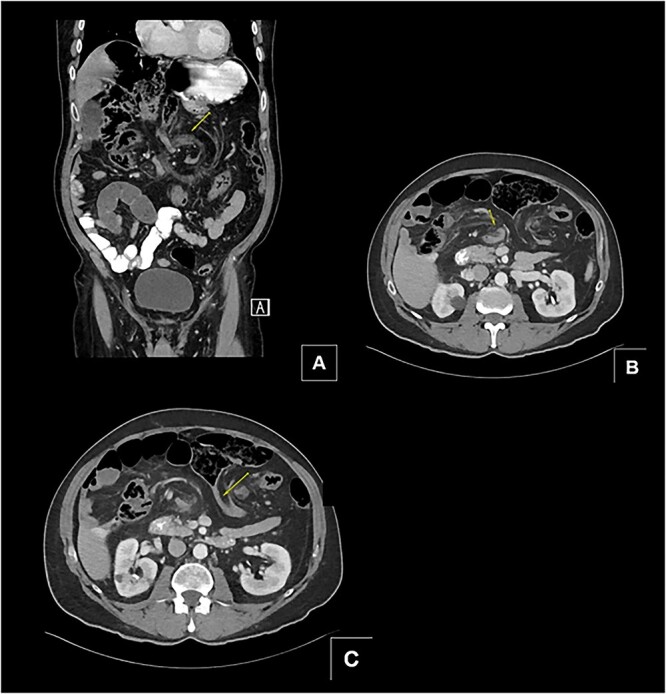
(A and B) Two separate CT images showing mesenteric swirling in distal small bowel and colon. (C) CT image showing severe luminal narrowing of distal transverse colon.
